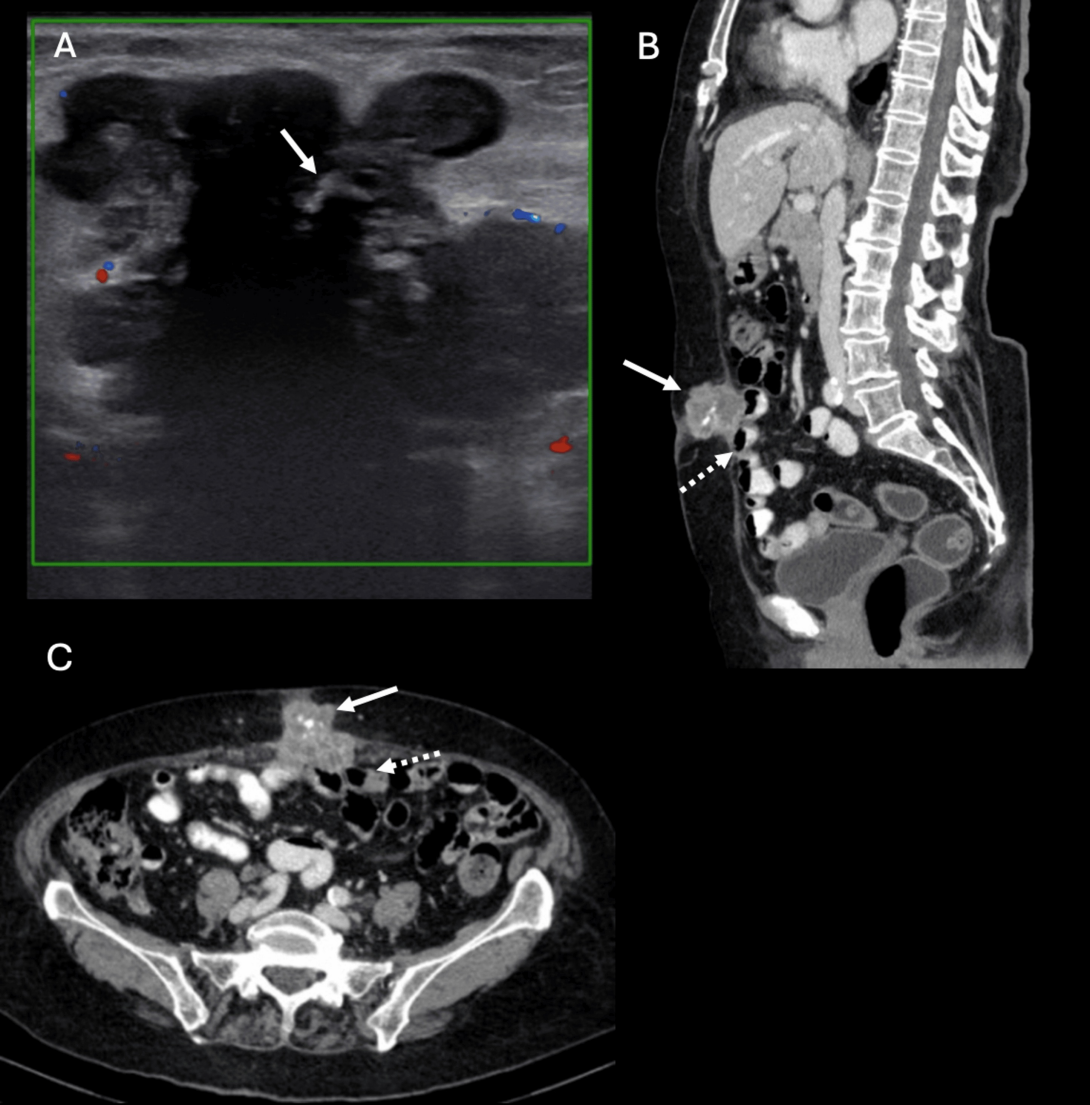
Ultrasound of the umbilicus (A) shows a well-defined, lobulated, hypoechoic umbilical lesion with internal calcifications (arrow, A), exhibiting minimal peripheral vascularity. Axial (arrow, B) and sagittal (arrow, C) views of contrast-enhanced CT of the abdomen further delineate the lesion's extension into the subcutaneous fat and preperitoneal space, compromising the fat plane with the adjacent ileum (dash arrow in image B and C).
